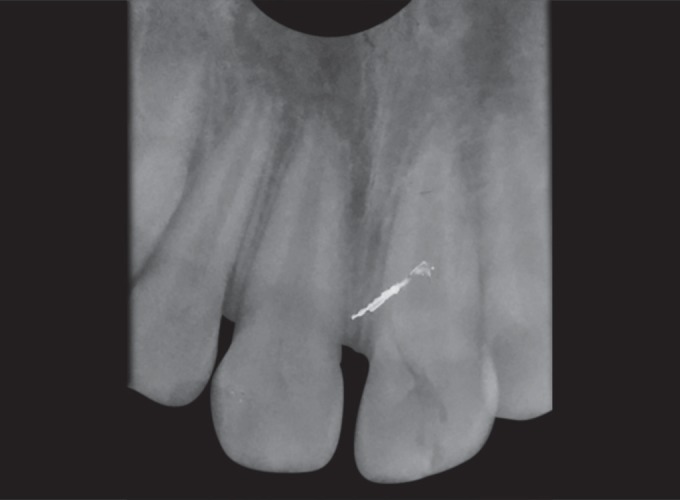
Periapical radiograph of the permanent left central incisor showing open apex, vertical fracture involving enamel, dentin, and pulp and extending up to 3 mm above the cervical line
